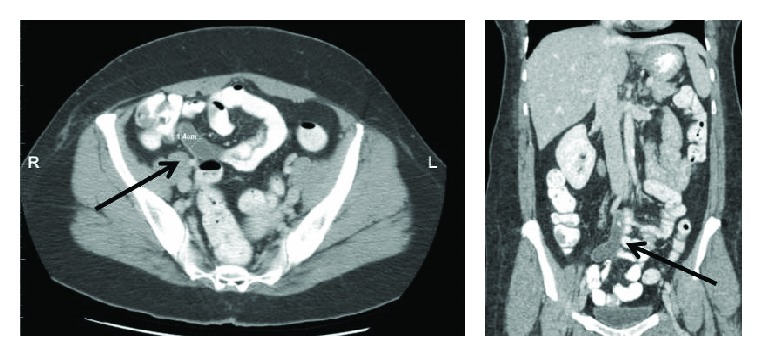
CT scan: appendix mucocele (arrow).
